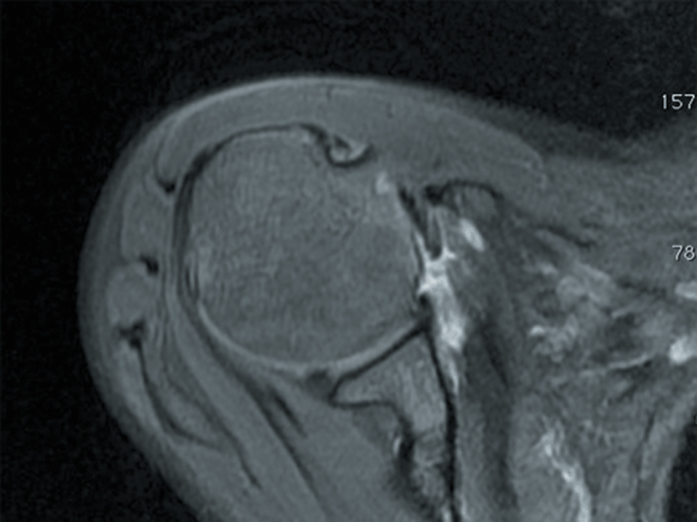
Axial MRI view of a patient with SSC tendon tear.
